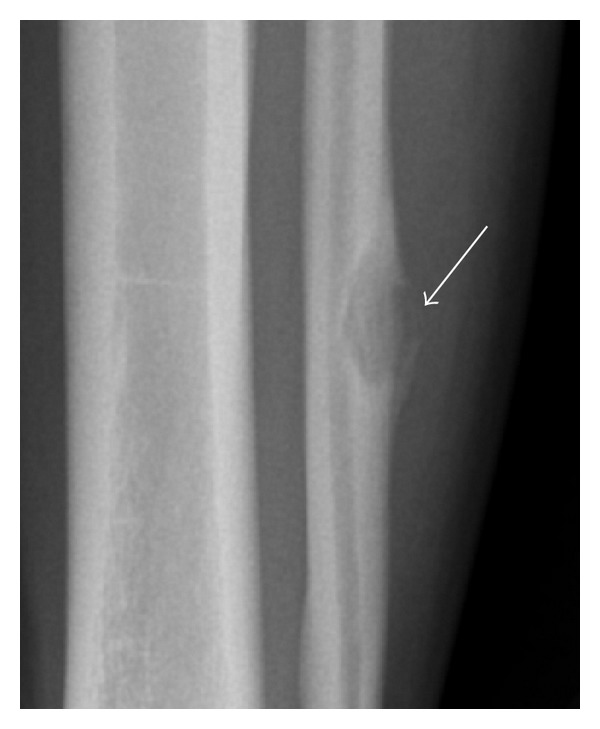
AP radiograph of the distal left fibula shows a lytic lesion with narrow zone of transition within the fibular cortex. There is periosteal elevation and a thin rim of bone surrounding the lesion (arrow).
